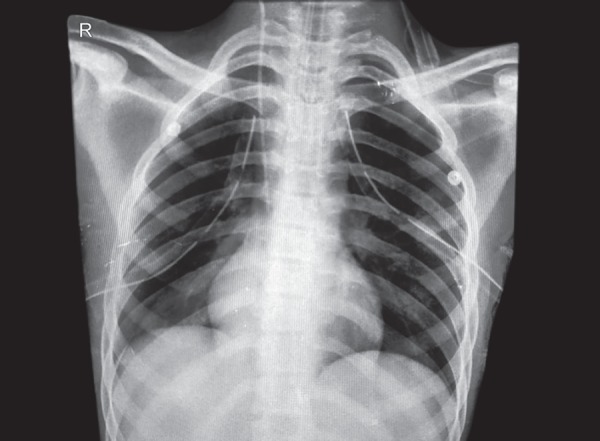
Chest X-ray AP view showing bilateral intercostal draining chest tubes
